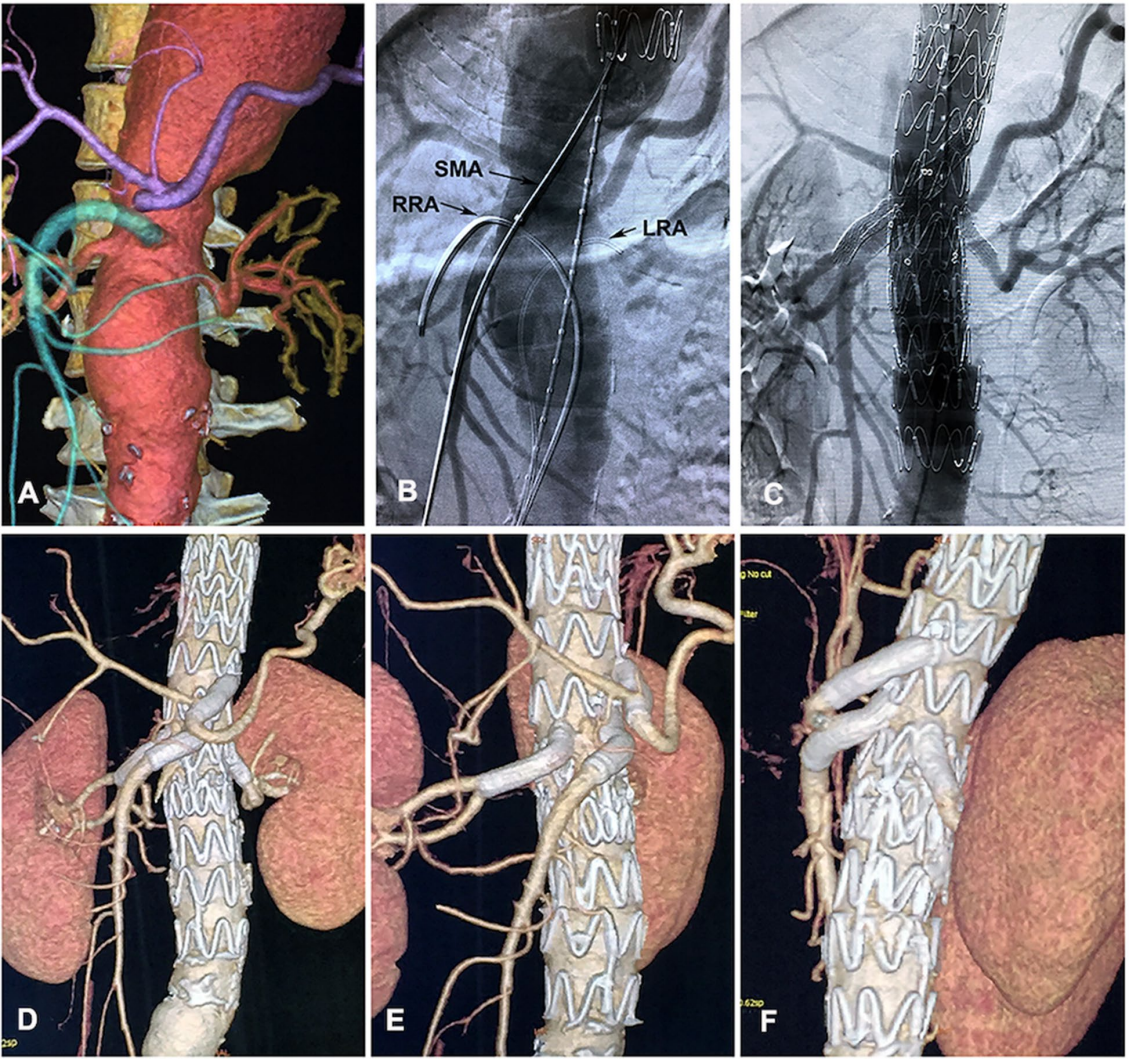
Pre-, intra-, and postoperative image of implantation of the Surgeon- modified fenestrated stent graft (PMSG) with 4 branches reconstruction. A Preoperative CTA of four visceral arteries. B Intraoperative angiography demonstrates catheterization of three visceral arteries. C Intraoperative angiography demonstrates implantation of PMSG with 4 branches reconstruction. D, E, F Postoperative CTA of 4 branches reconstruction in sagittal, right anterior oblique and left anterior oblique position, respectively
